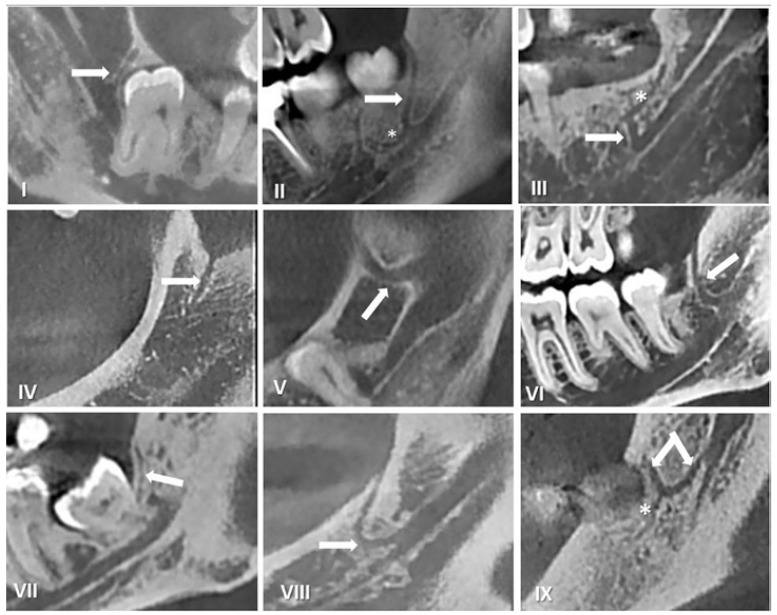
Different configurations of the retromolar canal observed on CBCT scan.
